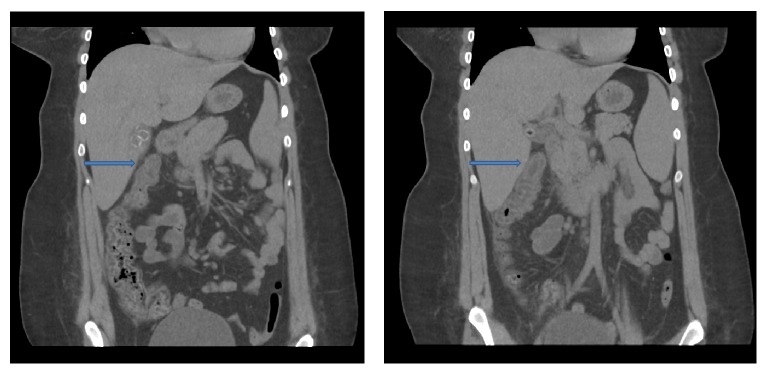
CT abdomen after C1 INH treatment, demonstrating resolution of intussusception (arrow).
